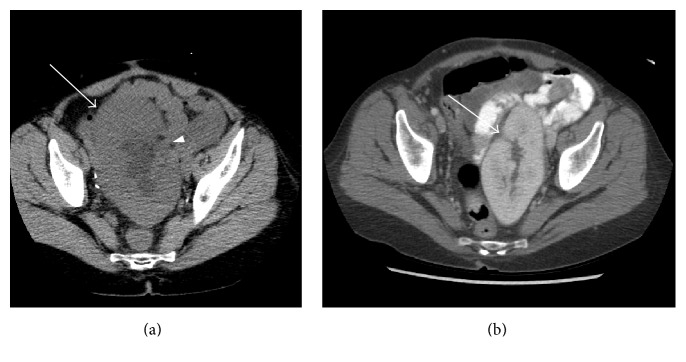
Noncontrast axial CT image through the pelvis (a) showed an enlarged left lower quadrant renal allograft (arrow) measuring 14.4 × 7.6 cm with moderate hydronephrosis and trace pelvic free fluid. Note that the hilum is directed laterally (arrowhead). This was a clear change in orientation when compared to a contrast enhanced CT scan obtained a year and half earlier (b) where the hilum was directed anteromedially (arrow).
